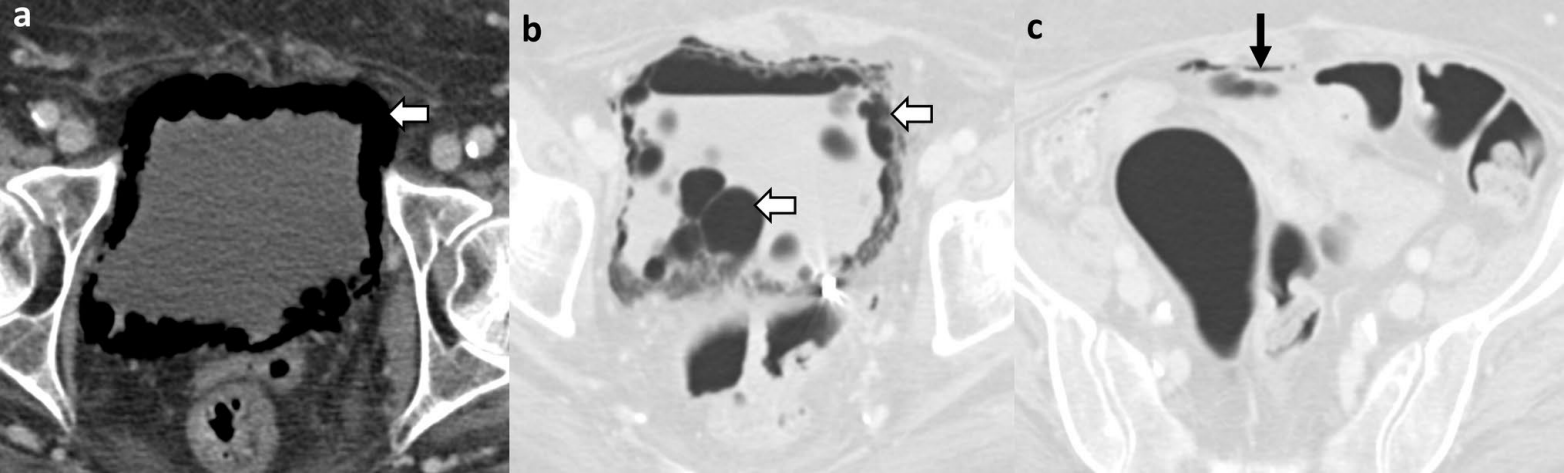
Emphysematous Cystitis. A 55-year-old diabetic patient with sepsis. CT images show extensive emphysematous cystitis (white block arrow, a, b) and trace pneumoperitoneum anteriorly (black arrow, c). Urinalysis was positive for urinary infection, and blood culture showed *Klebsiella pneumoniae*. The patient was managed with a Foley’s catheter and intravenous antibiotics but succumbed to sepsis
